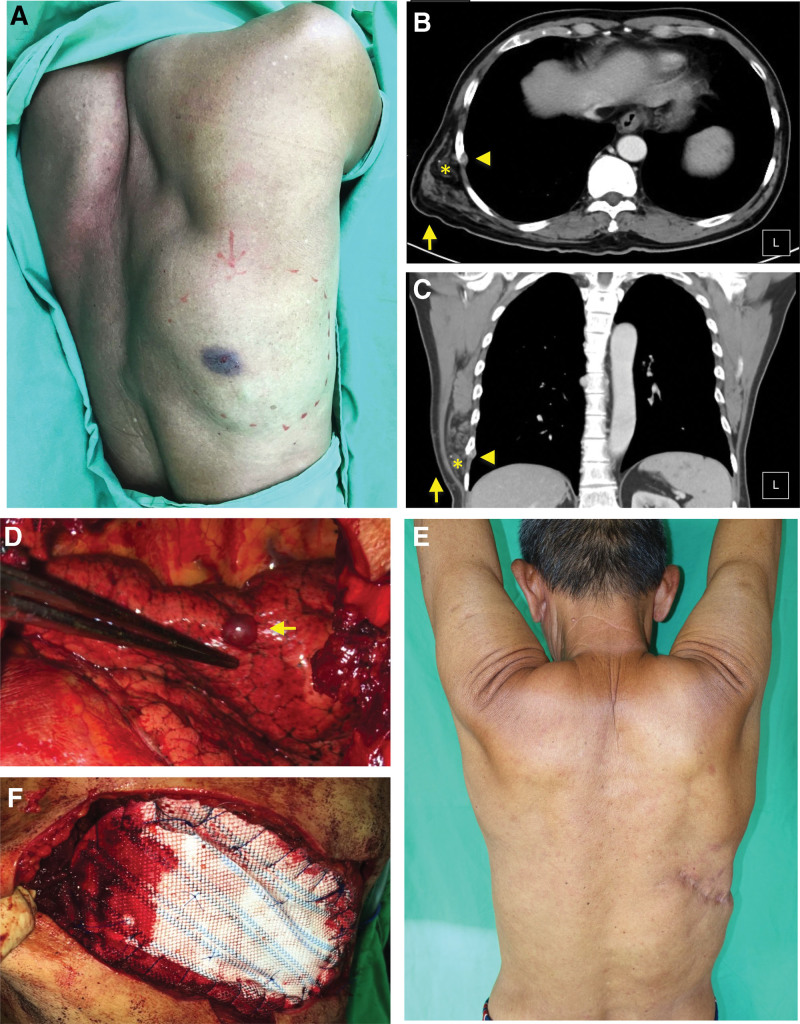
(A) A painful soft mass with a maximum diameter of 14 cm in the right lower back region with central bruising. (B and C) Contrast-enhanced CT scan images revealed a right chest wall mass (arrow) with calcification (yellow asterisk) and invasion to the right 7th, 8th ribs and pleurae (arrow head). (B) Axial view. (C) Coronal view. (D) Intraoperative image of hemorrhagic sponge-like vascular tissues were seen extensively involving the latissimus dorsi, ribs, pleurae and lung parenchyma (yellow arrow). (E) Intraoperative image of the polypropylene mesh for rib and pleura defects. (F) The follow-up results at 3 years postoperatively. No functional disability was observed. CT = computed tomography.
